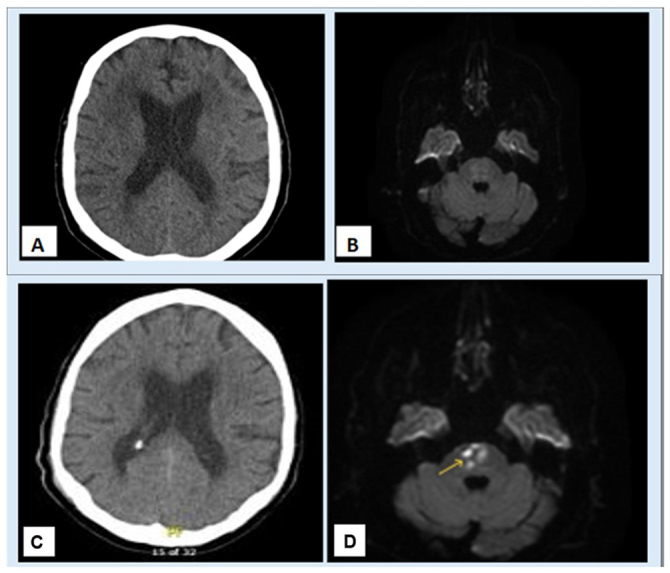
(A) Non-contrast computed tomography of the brain at initial presentation, showing no hemorrhage. (B) Magnetic resonance imaging (MRI) of the brain at initial presentation, showing no acute infarct. (C) Non-contrast computed tomography of the brain obtained during second presentation, showing no acute hemorrhage. (D) DWI MRI of the brain obtained during second presentation, showing acute pontine infarct.
